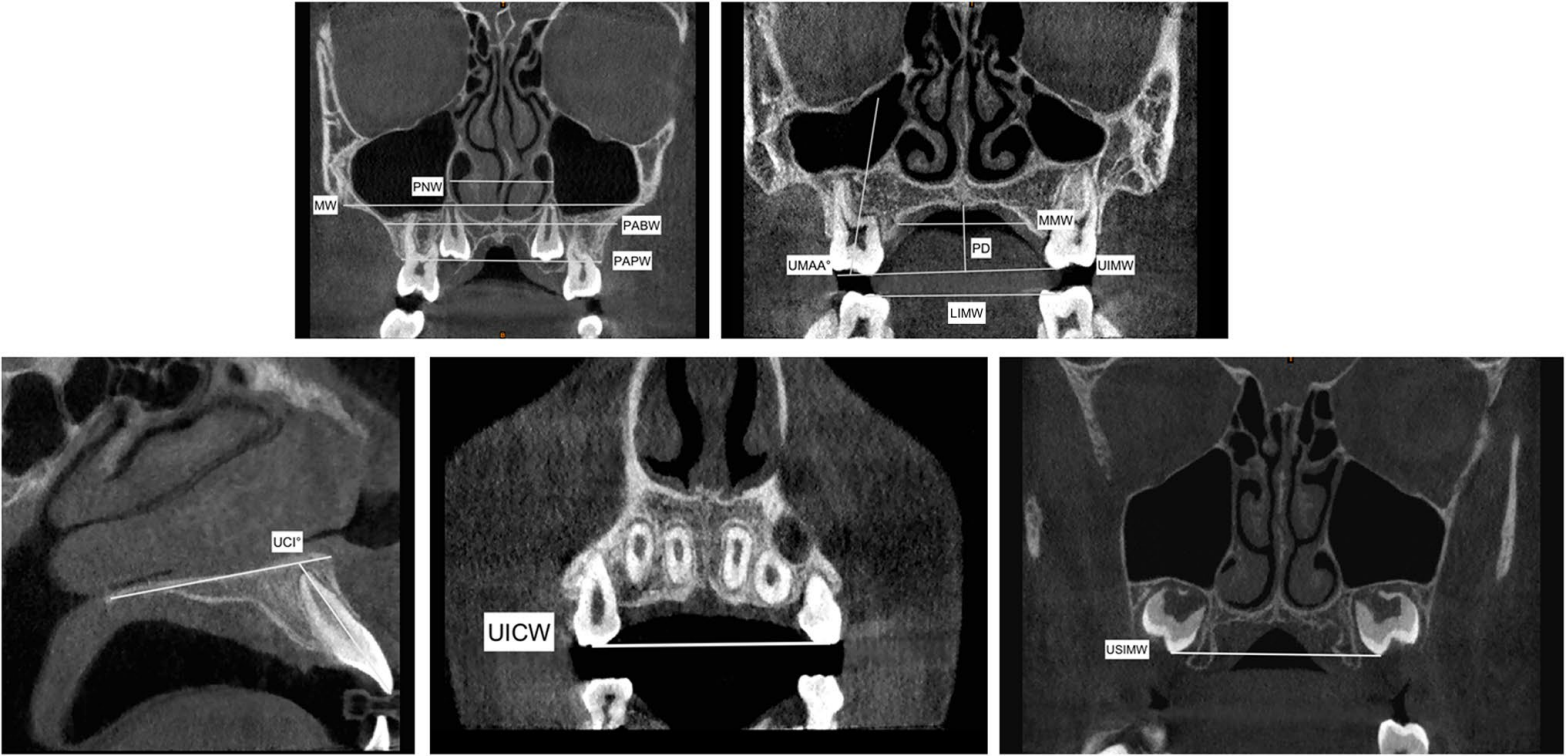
Representation of the skeletal and dentoalveolar measurements used in the present study evaluated on the CBCTs coronal and sagittal view
